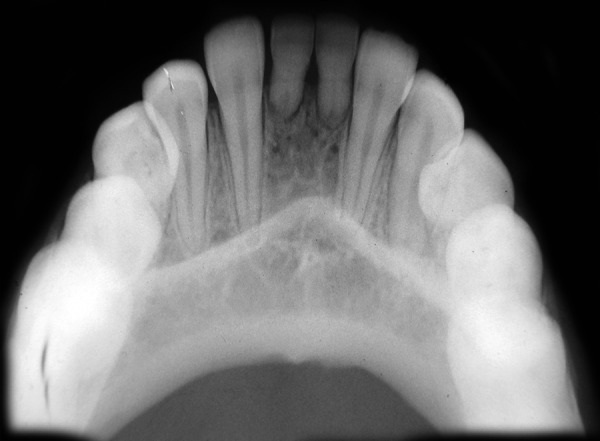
Mandibular occlusal radiograph showing bilateral absence of permanent mandibular central incisors
